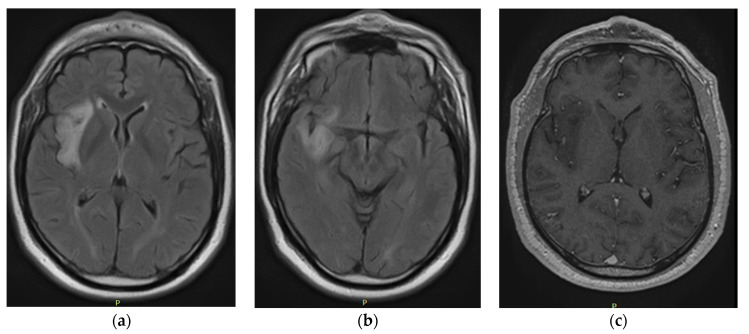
February 2020: iRM FLAIR axial (a,b) and T1 gadolinium (c).
